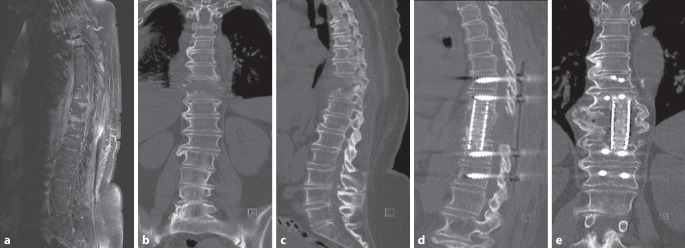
A 69-year-old patient with pyogenic spondylodiscitis at T9–10 underwent posterior debridement and interbody fusion, with a vancomycin-loaded antibiotic carrier applied intraoperatively. a–c Preoperative MRI and CT scans show destruction of the T9–10 intervertebral disc and adjacent vertebral bodies. d,e At 3 months postoperatively, the patient achieved Bridwell grade 2 fusion. The cage remained well positioned, and notable new bone formation within the fusion site suggested the onset of osseous healing
